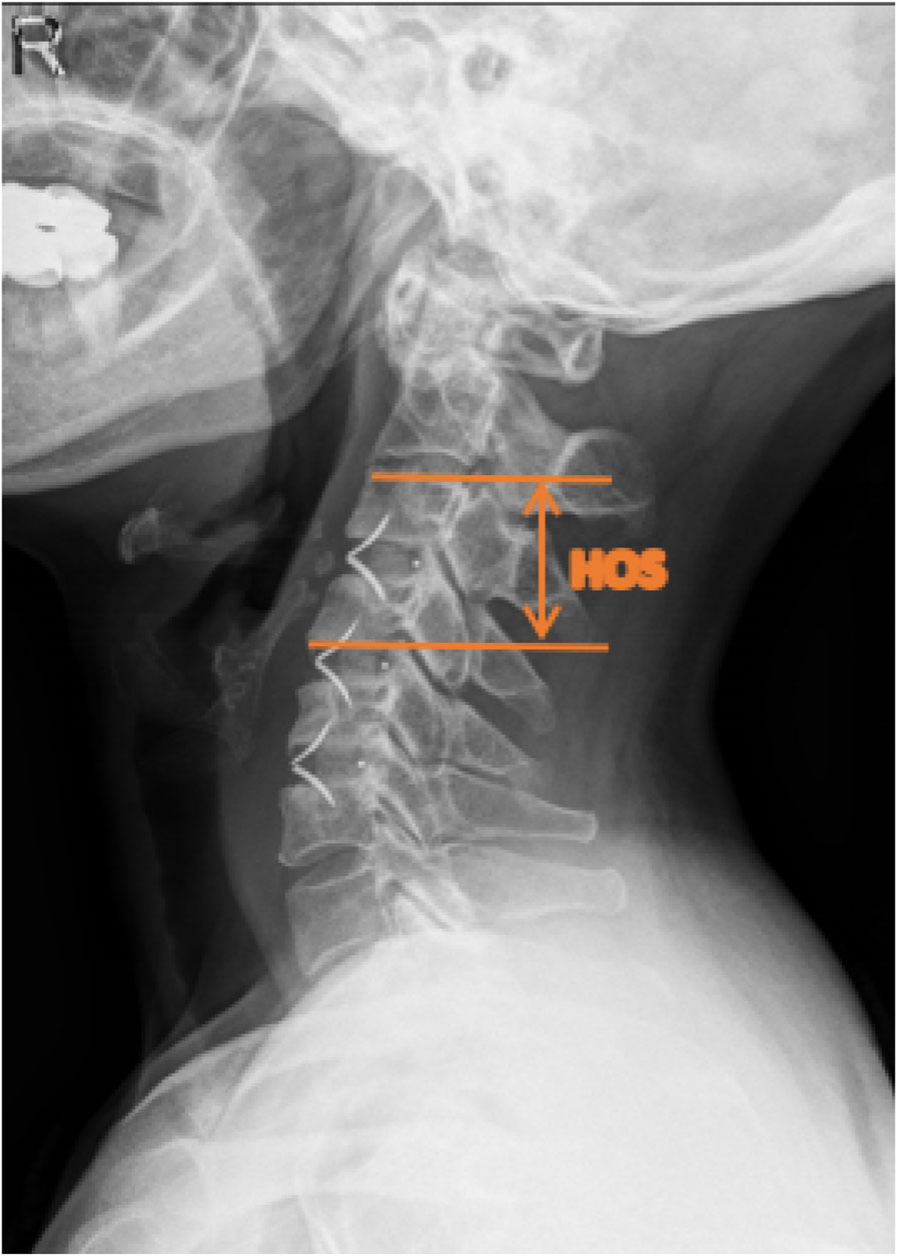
Abbreviations: HOS (height of operation segment) is the distance between the midpoint of the upper endplate of the upper vertebral body and the midpoint of the inferior endplate of the upper vertebral body on the lateral plain film of the cervical vertebra in the neutral position. For patients with double-segment or three-segment cervical surgery, the average height of the cervical spine was taken as the average
